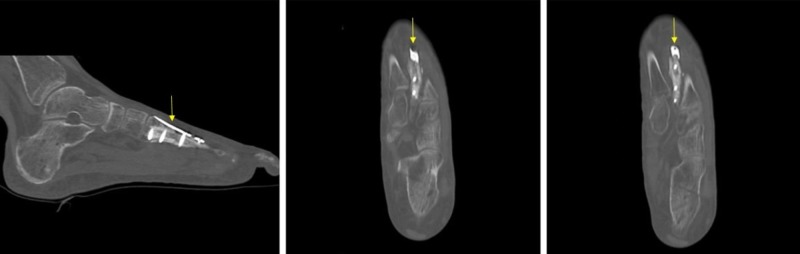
Post-operative computed tomography (CT) scan after revision open reduction and internal fixation with plate and screws.
